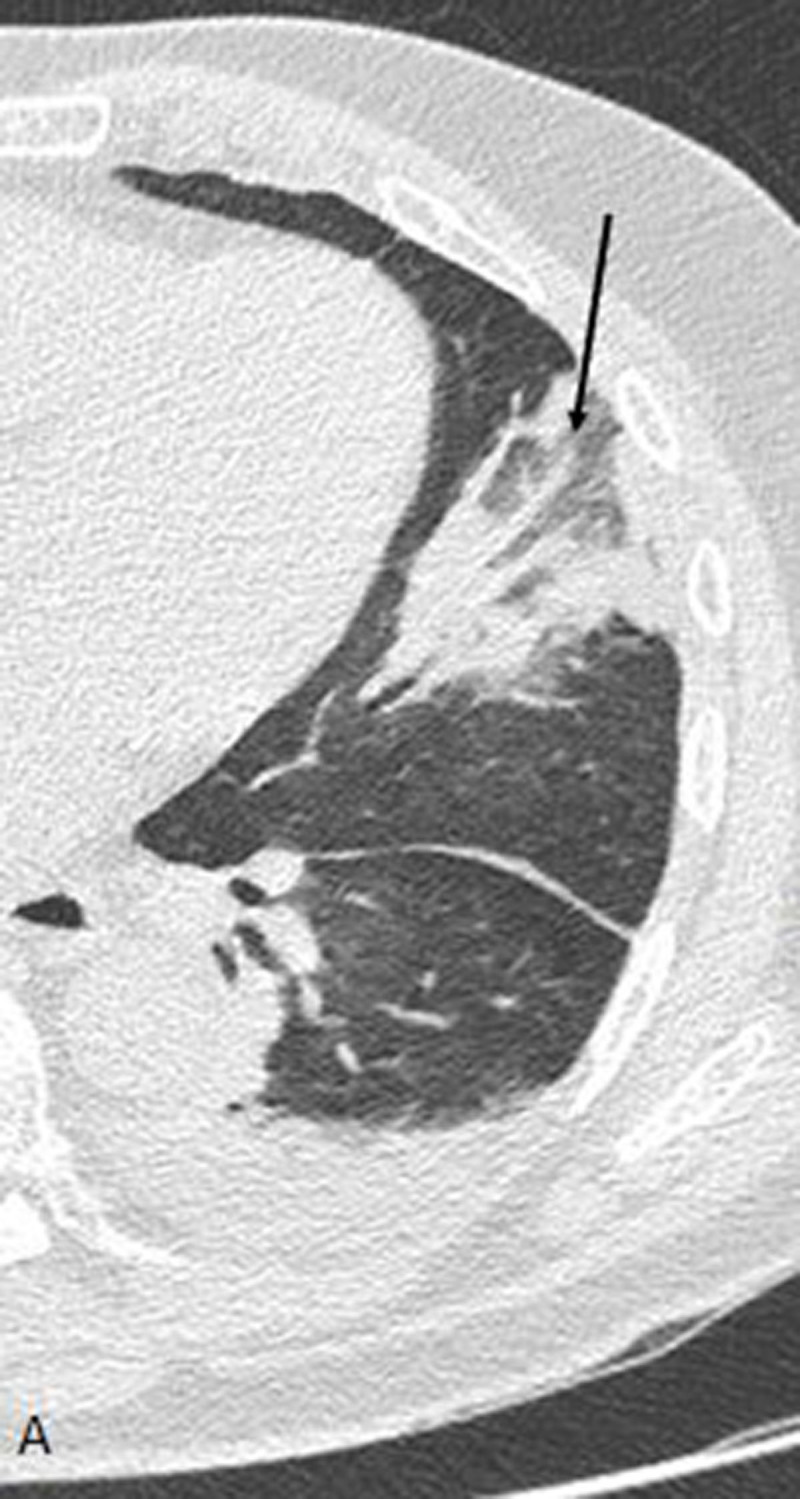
The combination of these CT findings was highly suggestive of an acute peripheral pulmonary embolism and was confirmed by pulmonary scintigraphy (Figure 3, oblique posterior left view (A) and oblique anterior left view (B); the white arrow shows a perfusion defect in the sub-segmental left inferior lingula artery territory).
